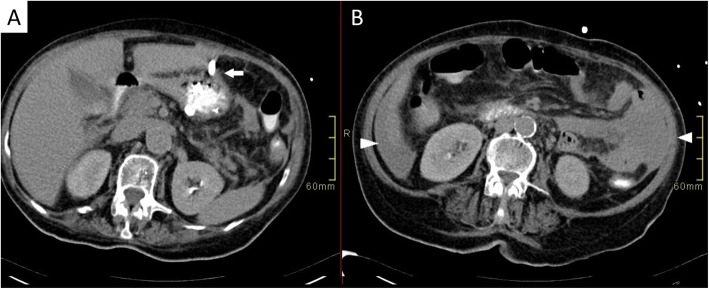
CT scan of the abdomen was performed 24 h after the placement of the new PEG demonstrated no water-soluble contrast leak from the newly placed PEG tube (Fig. 3 a: white arrow). Small volume ascites and limited peritonitis is noted secondary to the removed ruptured PEG tube (Fig. 3b: arrow tip)
